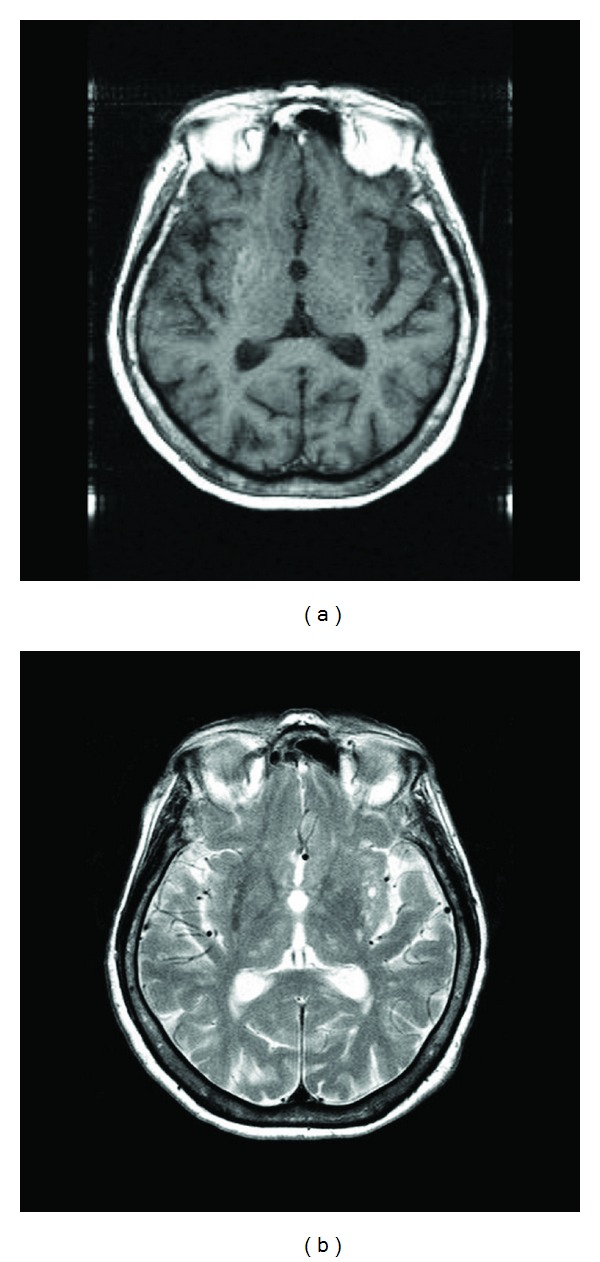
(a) MRI scan showing a hyperintense lesion on T1-weighted image. Arrow corresponds to the right striatum. (b) MRI scan showing a low signal intensity on T2-weighted image.
